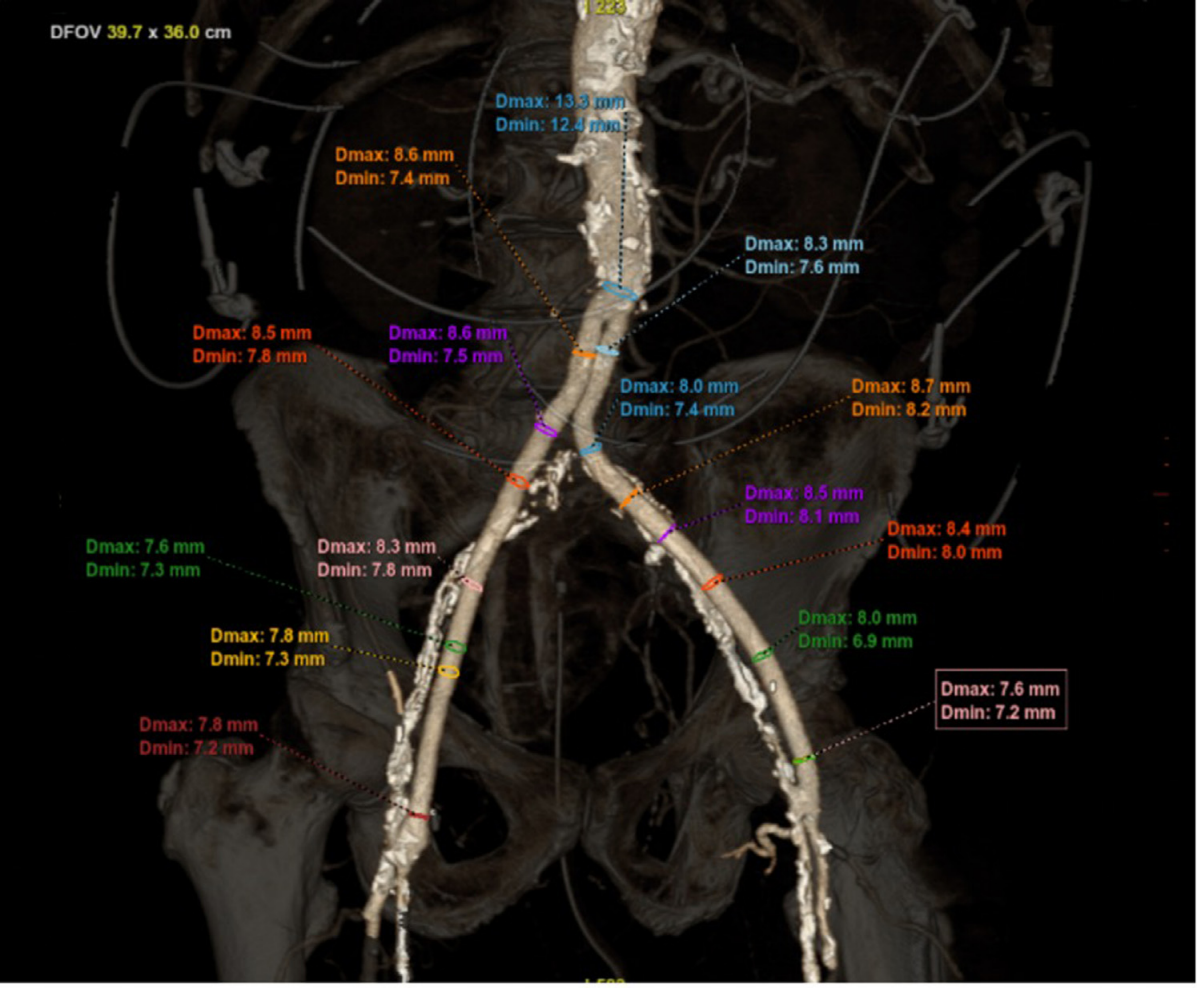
Preprocedural computed tomography angiography with reconstruction of the iliofemoral vessels, withbilateral aortofemoral bypass grafts demonstrated. DFOV, display field of view; Dmax, maximum diameter; Dmin, minimum diameter.
